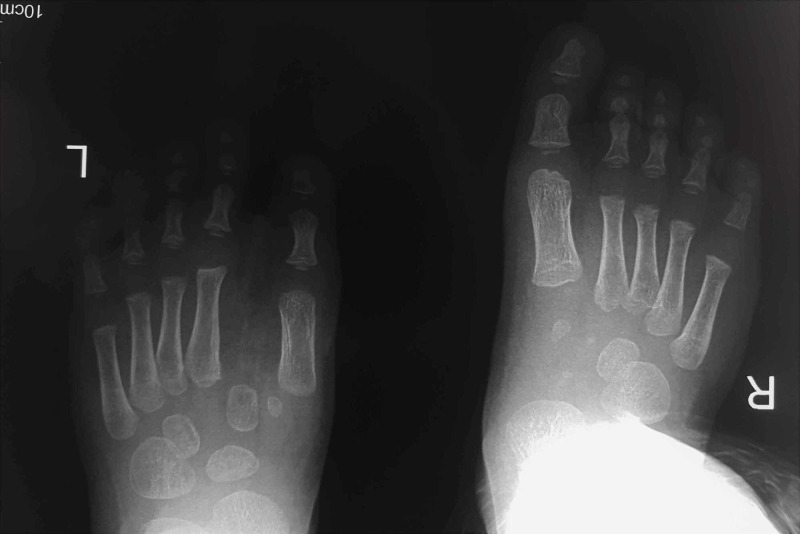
Two-year follow-up X-ray (XR) Two-year follow-up XR where a mild widening of the middle foot is apparent.
